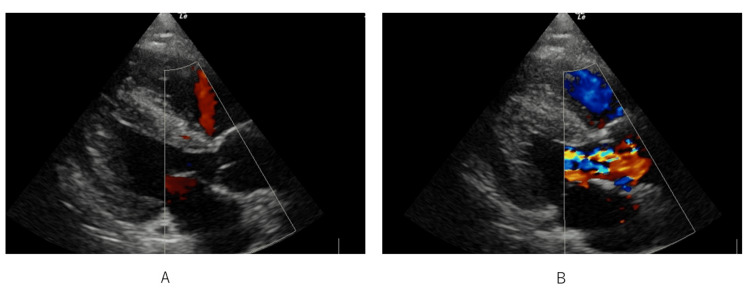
Trans-thoracic echocardiography upon admission to the ICU Trans-thoracic echography indicated a normal left ventricular ejection fraction (>60%) and normal diastolic function. A small amount of pericardial effusion was confirmed. A: Diastolic phase. B: Systolic phase.
